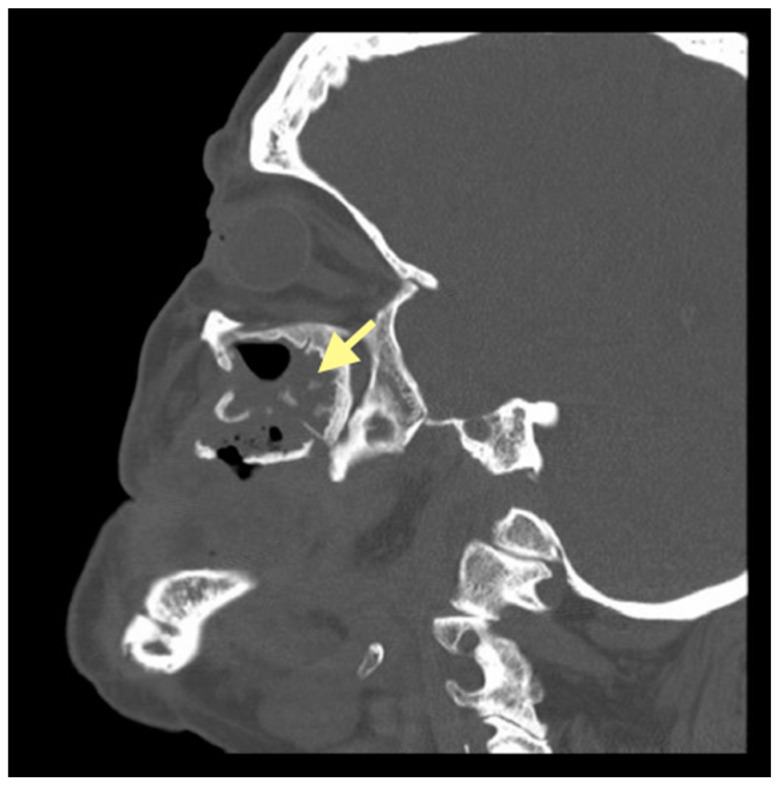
CT of the head and facial bones (19 March 2025) before the last operation (sequestrectomy of the lower and upper jaws; antrotomy performed).
